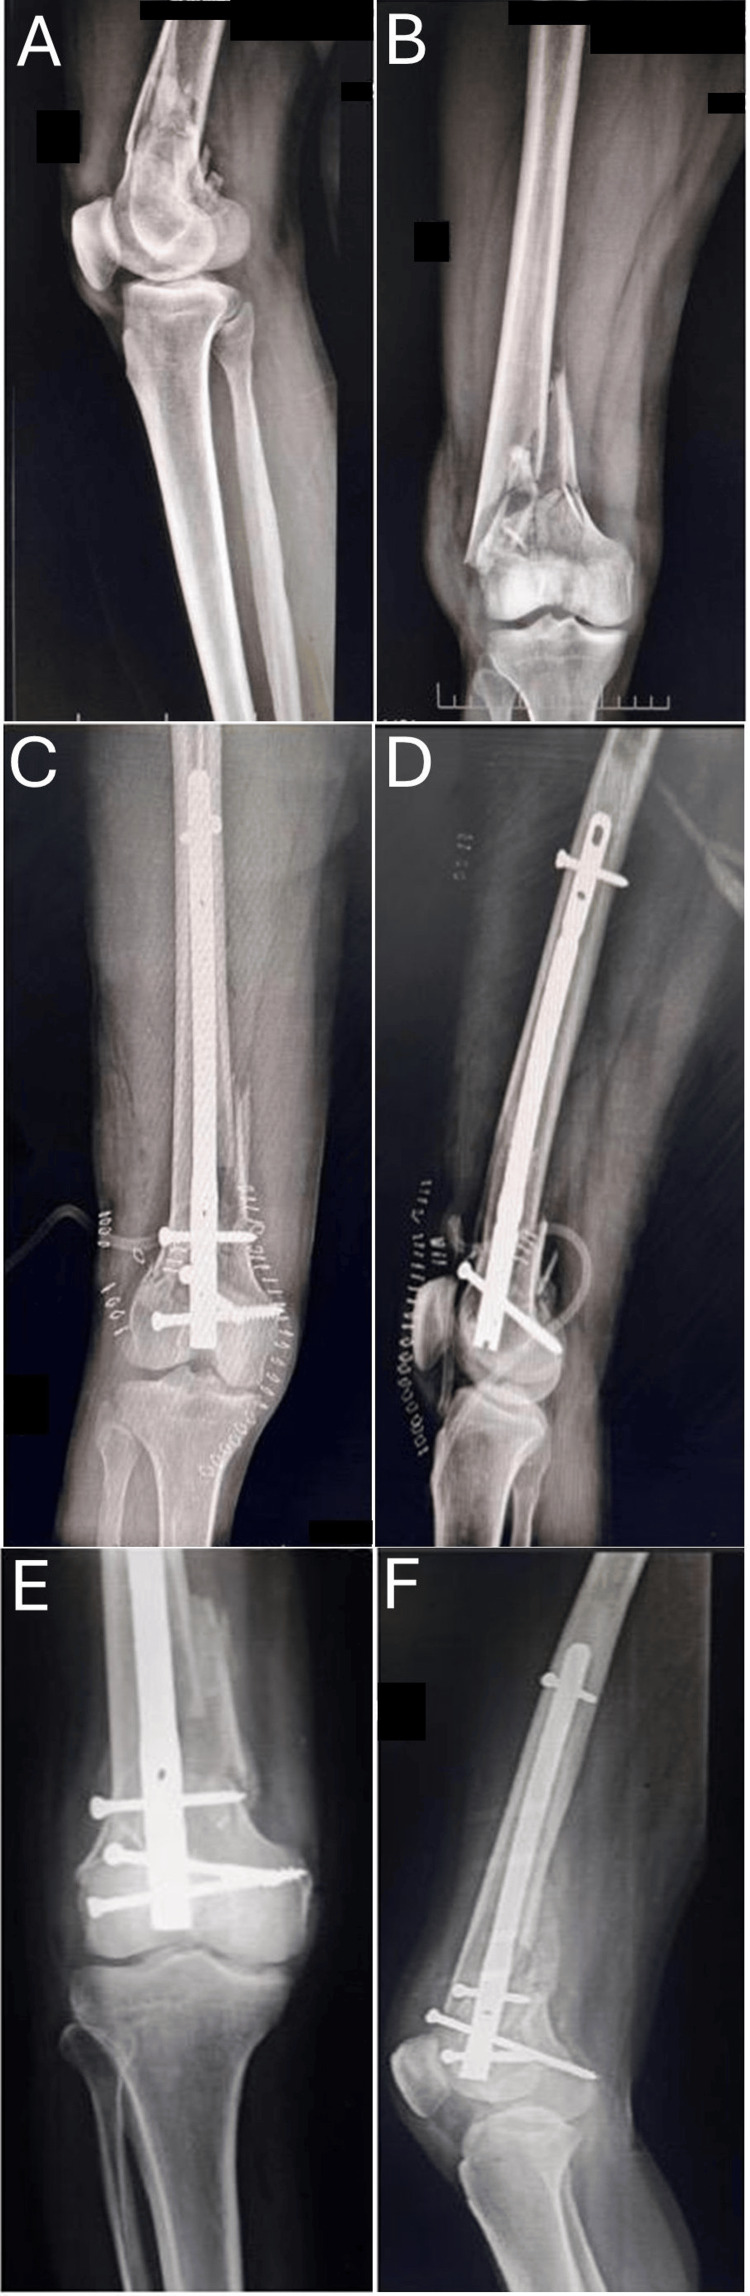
Multifunctional retrograde femoral nail fixation - image 2 (A) Preoperative anteroposterior radiograph of AO C2 type fracture. (B) Preoperative lateral radiograph of AO C2 type fracture. (C) Postoperative (immediate) anteroposterior radiograph. (D) Postoperative (immediate) lateral radiograph. (E) Postoperative (one-year follow-up) anteroposterior radiograph. (D) Postoperative (one-year follow-up) lateral radiograph
